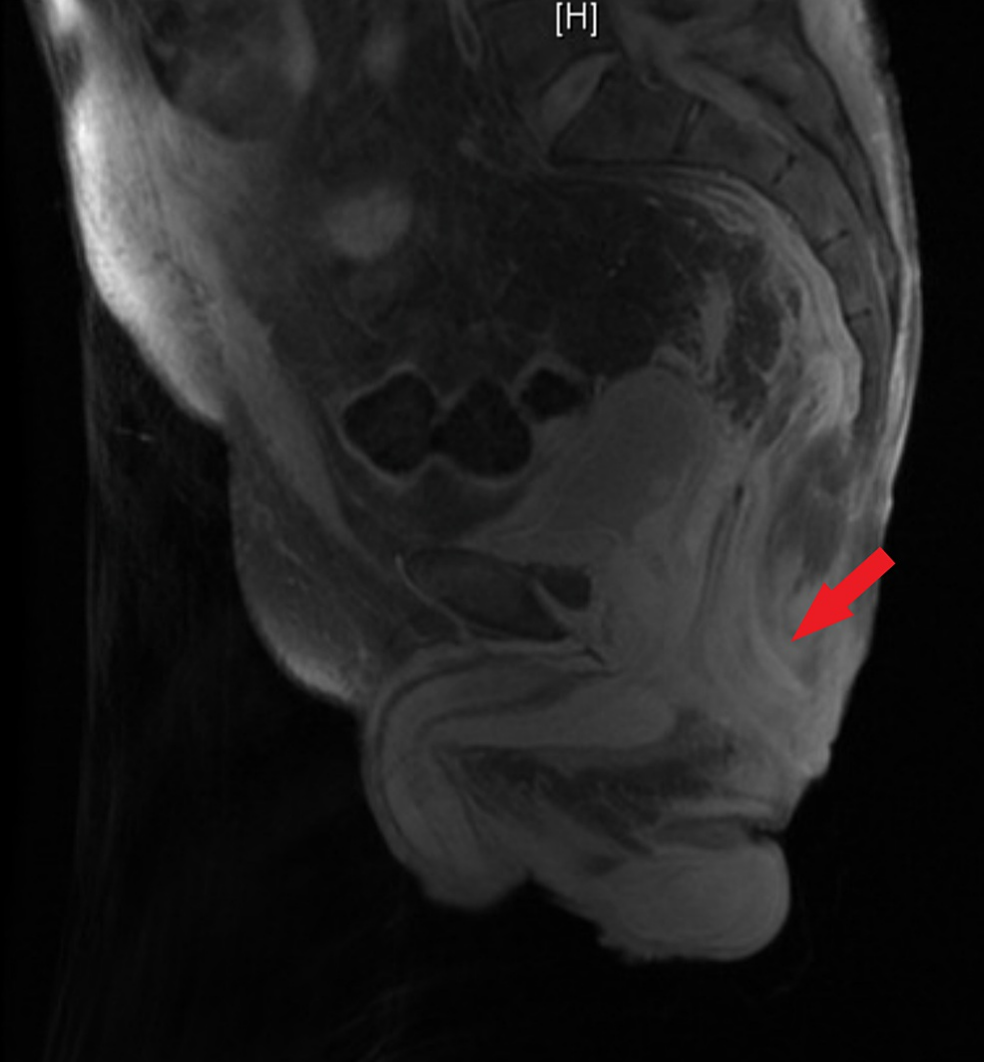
Sagittal view of pelvic MRI. Red arrow indicates the rectal stump without any visible abscess or fistula. MRI, magnetic resonance imaging
